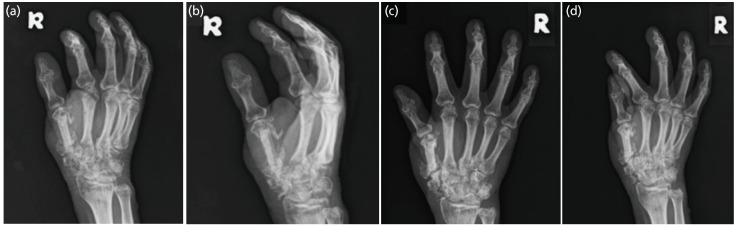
(a and b) Radiographs taken at three and (c and d) six months post-surgery showing progressive integration and union of the autograft.
